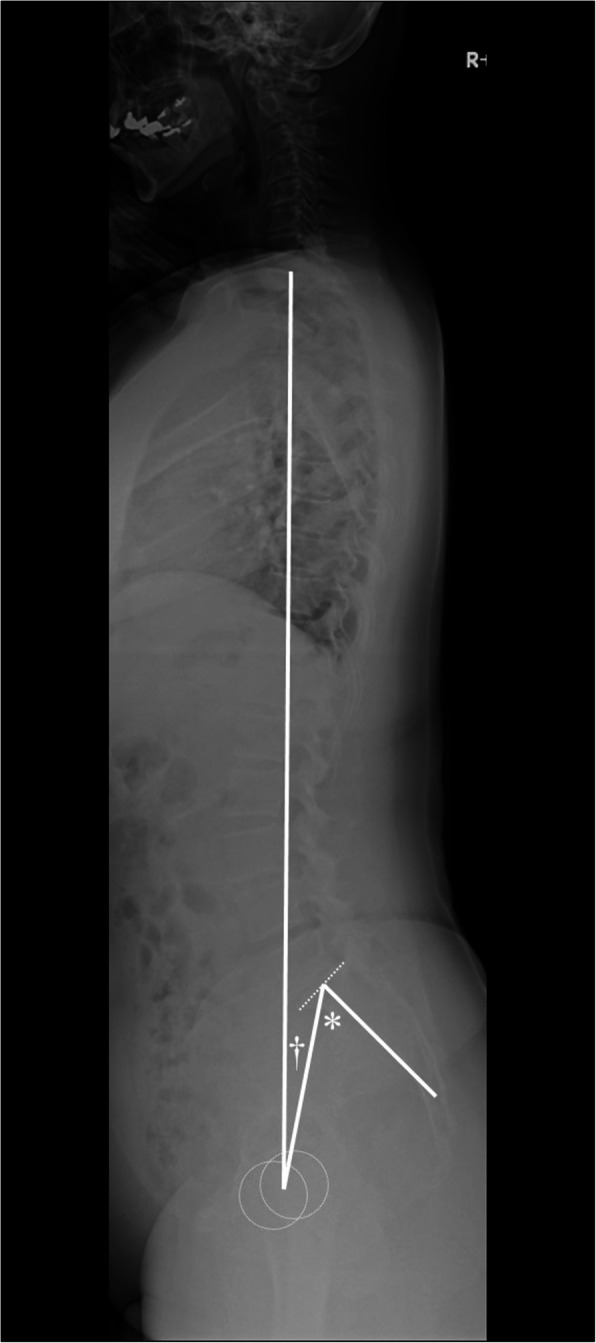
A radiograph was used to evaluate the pelvic incidence (*) and T1 pelvic angle (T1PA, †) of a patient with a global sagittal spinal deformity [6, 19]. T1PA is defined as the acute angle by the intersection of a line from the centre of the T1 vertebral body to the femoral heads and a line from the femoral heads to the centre of the superior sacral end plate. This interplay suggests useful information from both the sagittal vertical axis and pelvic tilt simultaneously to measure the geometry of global spinal deformity
